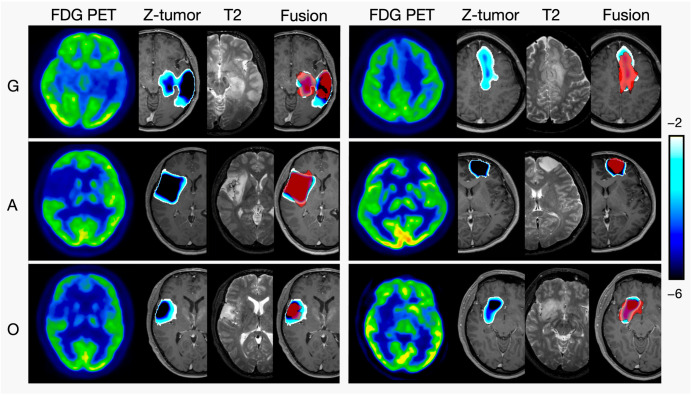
Representative cases showing ^18^F-fluorodeoxyglucose positron emission tomography (FDG PET), Z-tumor volume of interest (VOI), and T2-weighted images. Representative cases are shown for glioblastoma, isocitrate dehydrogenase (IDH)-wildtype (G), astrocytoma, IDH-mutant (A), and oligodendroglioma, IDH-mutant and 1p/19q-codeleted (O). The Z-tumor VOI was defined as a tumor-associated hypometabolic region with Z-scores < −2. Z-tumor VOI was fused with the T1-weighted image, and the color scale represents Z-scores from −6 to −2. The fusion image shows the VOI-T2 (red), corresponding to the T2-hyperintense region, overlaid on the Z-tumor VOI.
